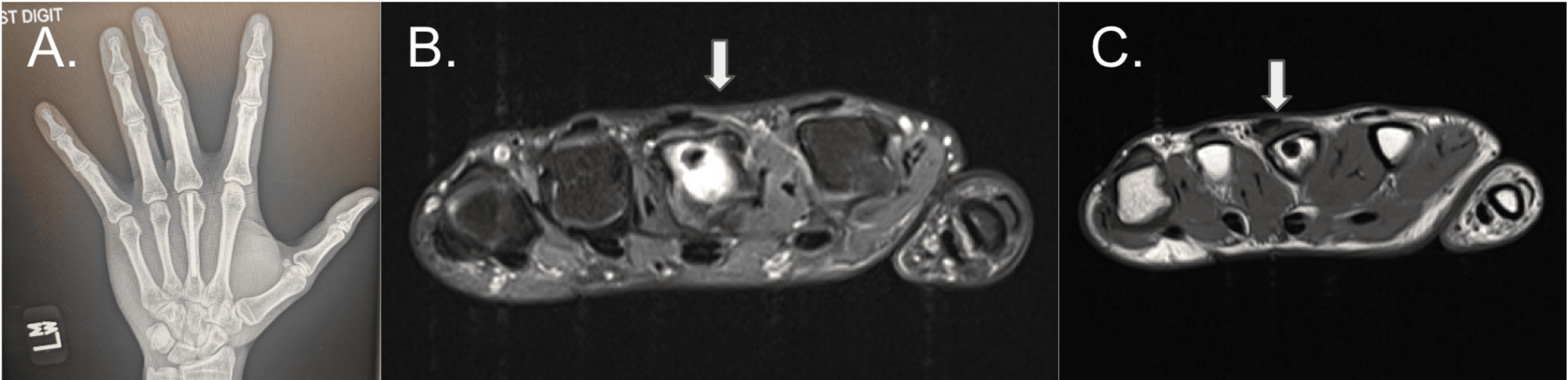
Patient imaging: (A) PA radiograph of the left hand demonstrating percutaneous screw fixation of the third metacarpal. (B) MRI of the left hand demonstrated concern for radial sagittal band disruption and (C) ulnar translation of the extensor tendon of the finger.
